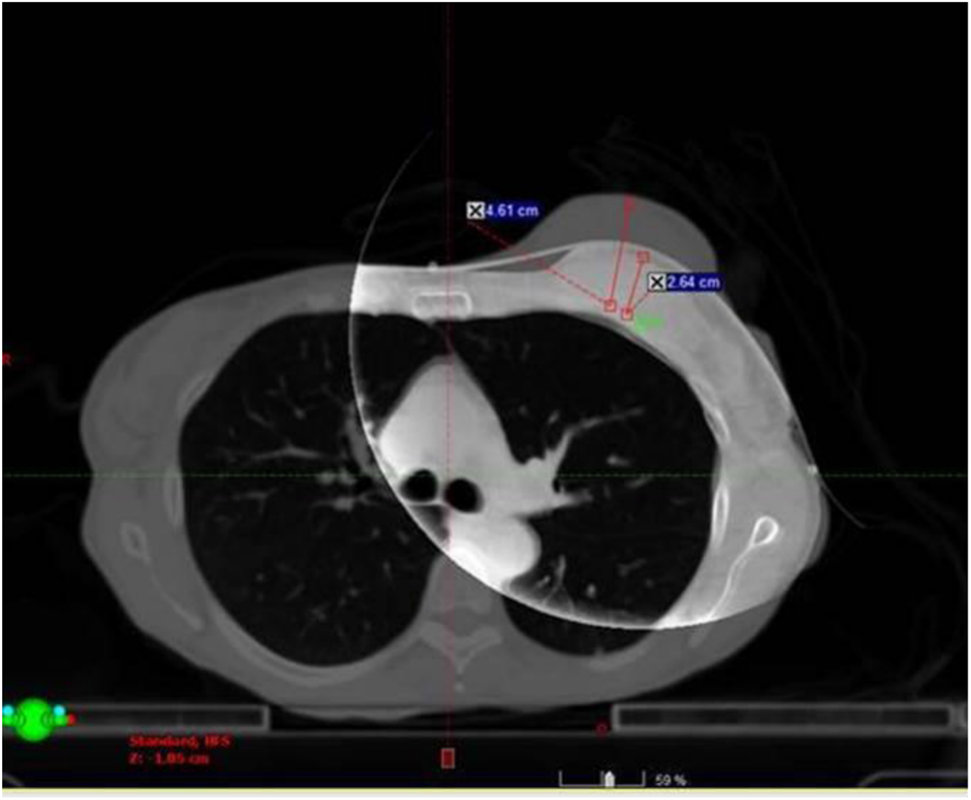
Cone beam computed tomography (CBCT) detected a clinically relevant change in breast tissue (2.0 cm) in an atypical patient with no surgery.
